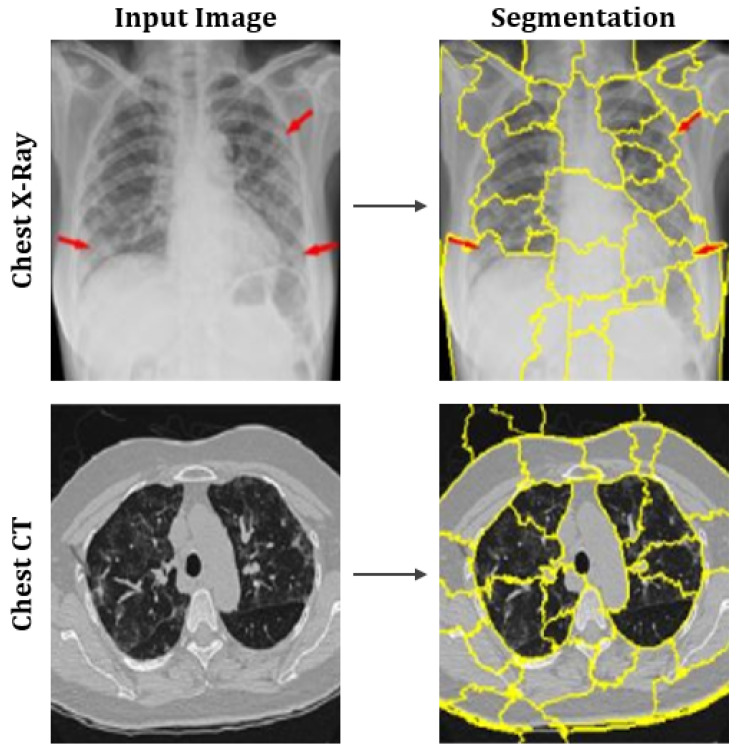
Representation of superpixels on sample images of chest X-rays and CT scans.
